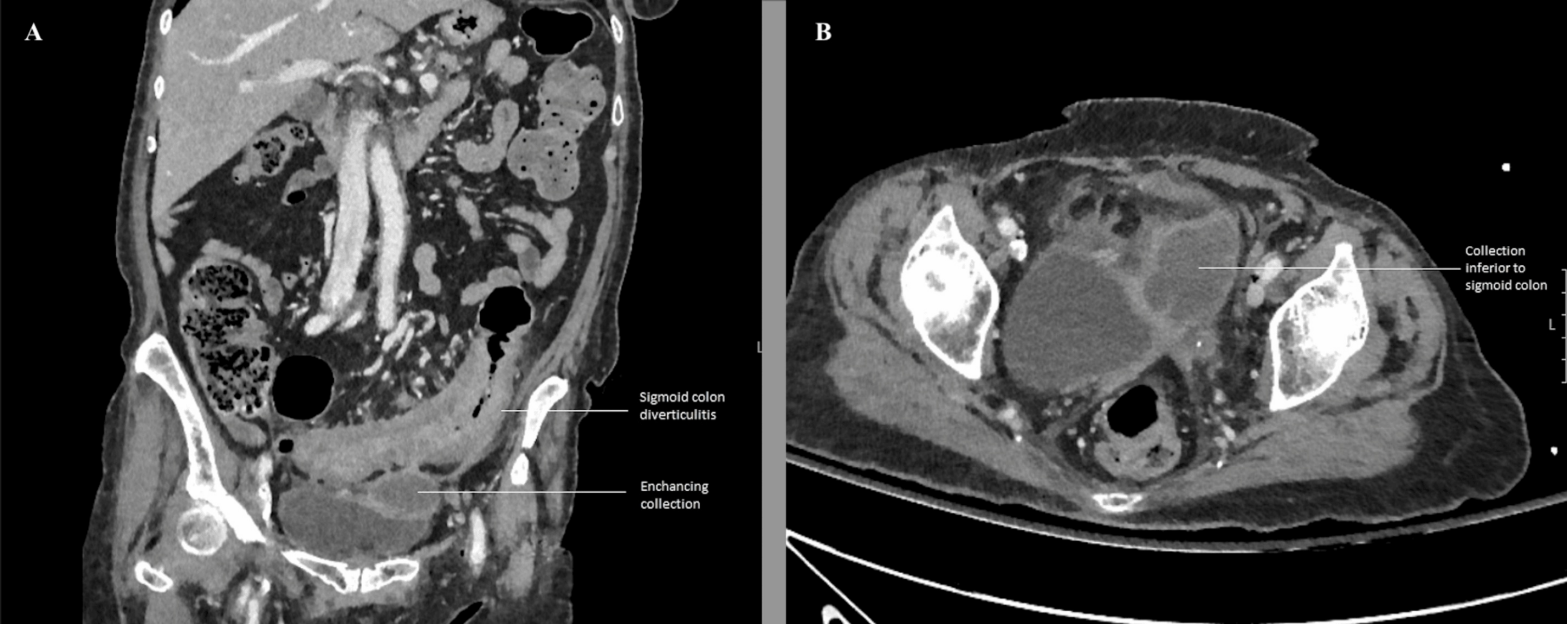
Computed tomography (CT) of the abdomen and pelvis showing signs of diverticulitis with collection (A) Coronal CT section showing sigmoid diverticulitis with enhancing collection; (B) axial CT section showing collection inferior to the sigmoid colon
